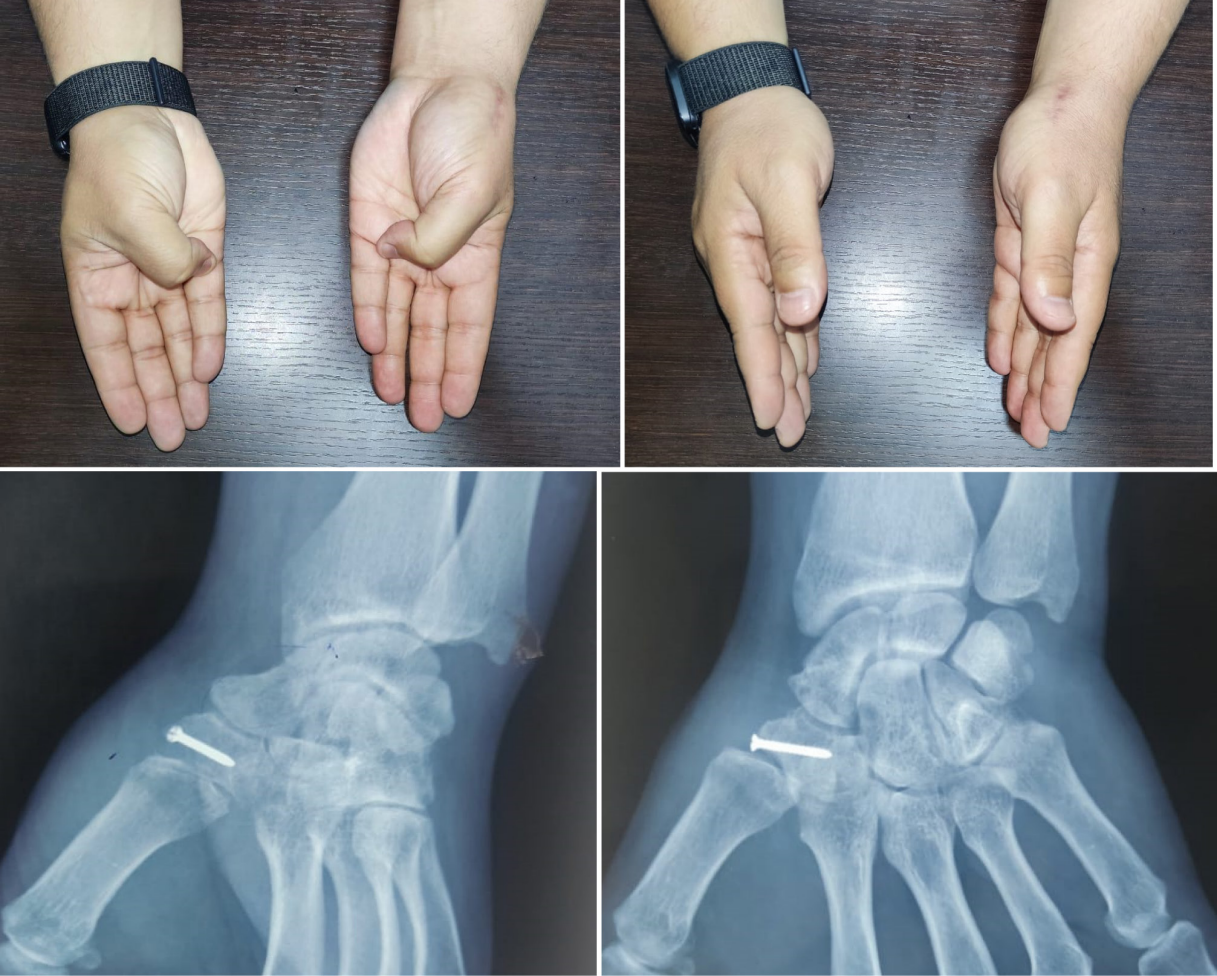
Clinical and radiological results at the end of the sixth week: full restoration of mobility of the thumb column with a kapendji score of 10, and radiography showing complete consolidation of the fracture.
